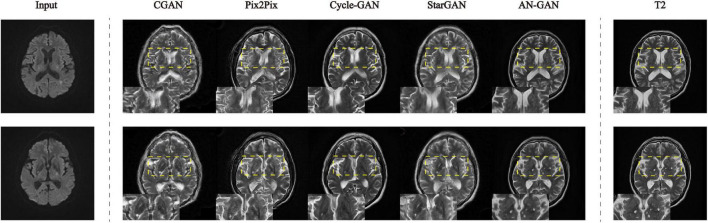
Experimental results of CGAN, pix2pix, Cycle-GAN, StarGAN, and AN-GAN in the test set. Synthesis of images from DWI to T2.
